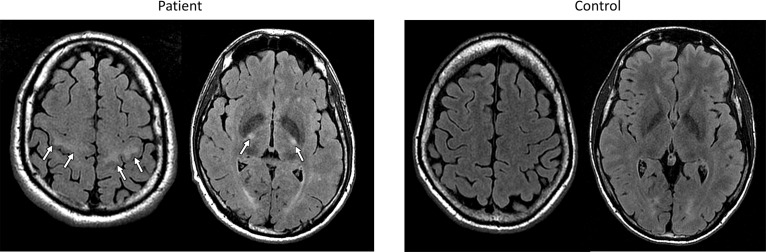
FLAIR MR images from an exemplary patient with ELA (left) and a control subject (right) evaluated in our center, showing bilateral hyperintensities of the corticospinal tract in subcortical white matter and posterior limb of capsula internal in the ELA patient.
